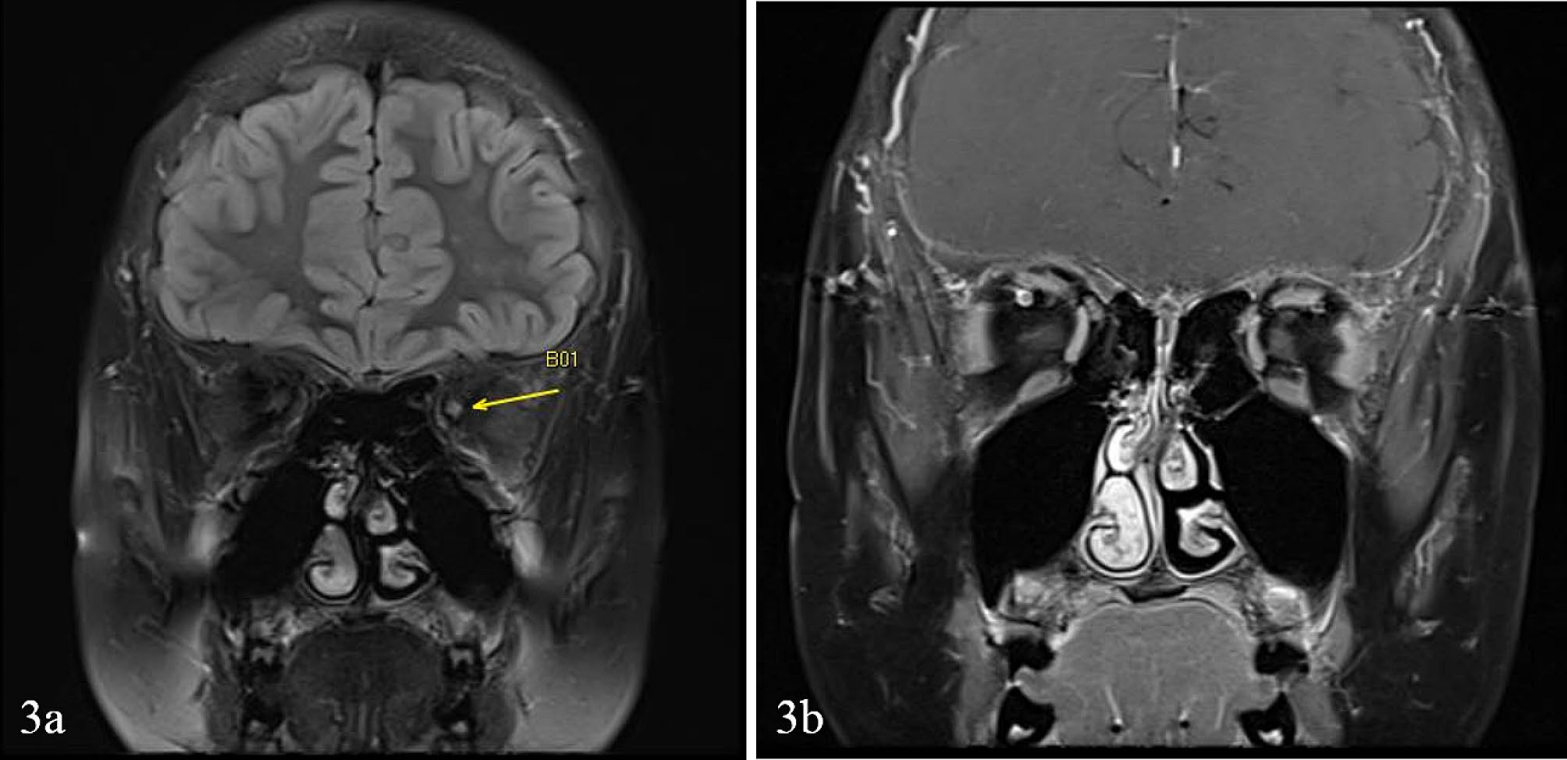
MRI imaging showing increased T2 signal intensity in the intra-orbital segment of the left optic nerve (Fig. 3a), with minimal enhancement noted on post-contrast T1 sequences (Fig. 3b)
